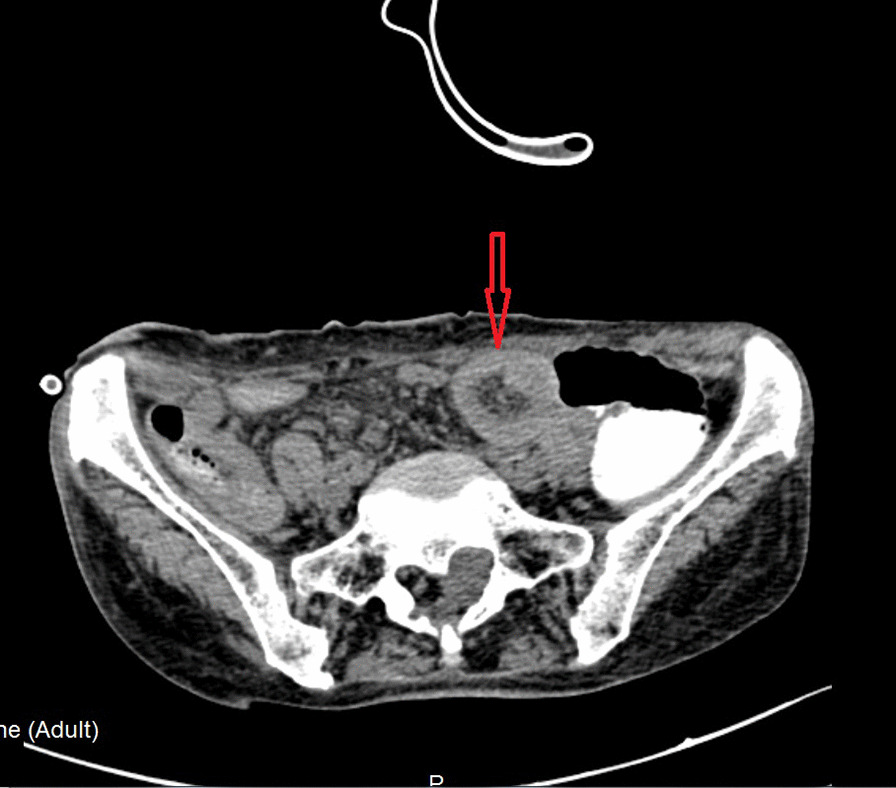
CT of the abdomen suggests intussusception(concentric circle sign)
